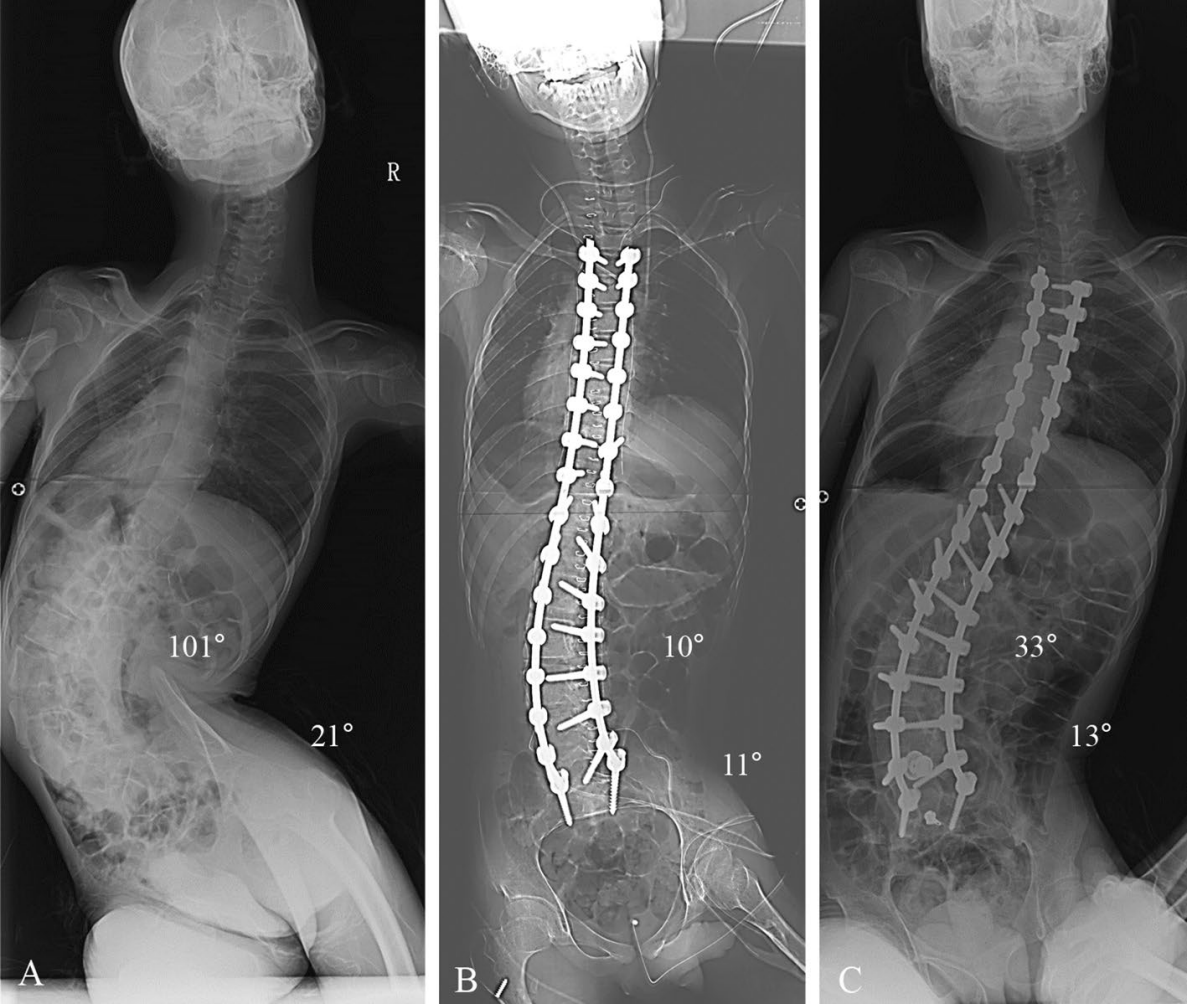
Deformity correction from T3 to S1 in patients with neuromuscular scoliosis (NMS). (A) A 16-year-old male patient who diagnosed with cerebral palsy (spastic quadriplegia) showed neuromuscular scoliosis. The whole-spine anterior–posterior image showed 101° of scoliosis deformity, and pelvic obliquity was 21° as measured by pelvic tilting angle. (B) The immediate postoperative radiograph showed 10° of the Cobb’s angle (90.1% of correction rate) and 11° of the pelvic tilting angle (47.6% of correction rate, which are not performed by spinopelvic fixation). (C) The 2-year follow-up Cobb angle was 33° and pelvic obliquity was 13°.
